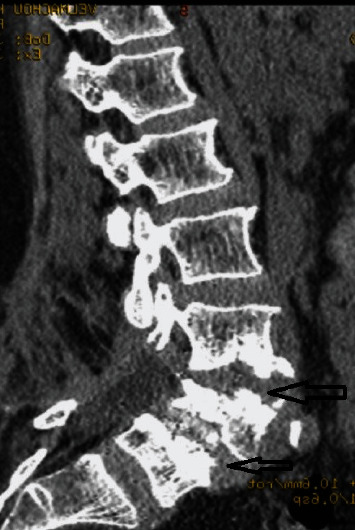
Preoperative CT-scan of a 71-year-old woman on admission.
